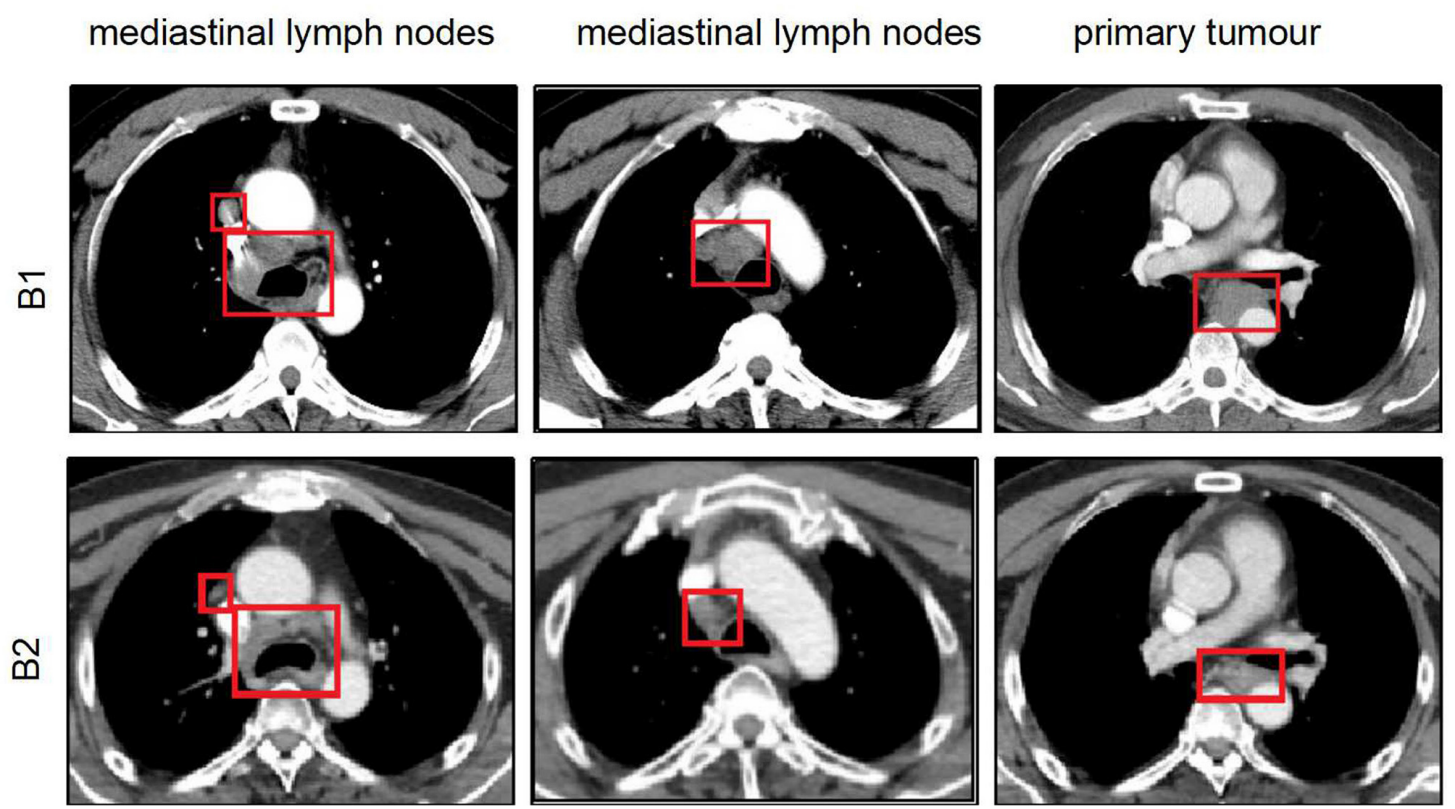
Comparison of chest CT scan between before and after treatment for the second patient. (B1) Chest CT showing that thickened esophageal wall and the enlarged lymph nodes in the mediastinum with an outcome of PD after patient underwent four cycles of chemotherapy. (B2) Chest CT scan representing that the primary tumor mass reduced in size and the enlarged lymph nodes in the mediastinum shrank or became loosened with an outcome of PR after anlotinib combined with radiotherapy and two cycles of chemotherapy. CT, computed tomography; PD, progressive disease; PR, partial progressive disease.
